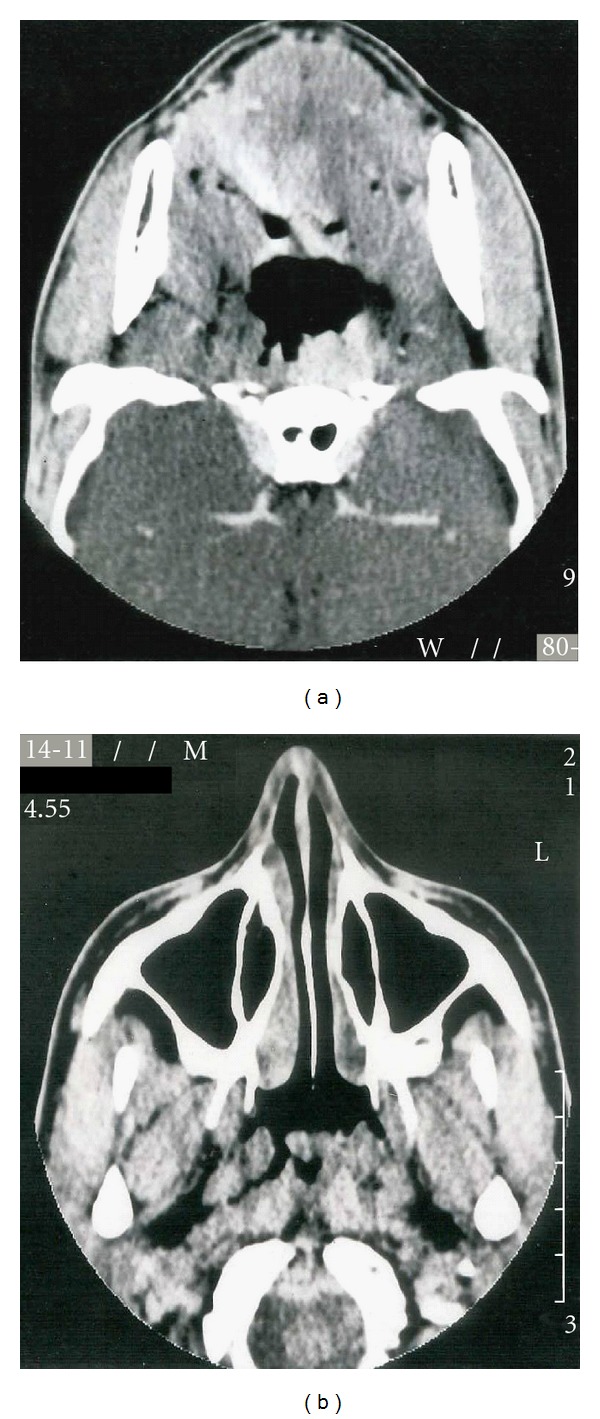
Facial CT: thickening of the left nasopharynx.
